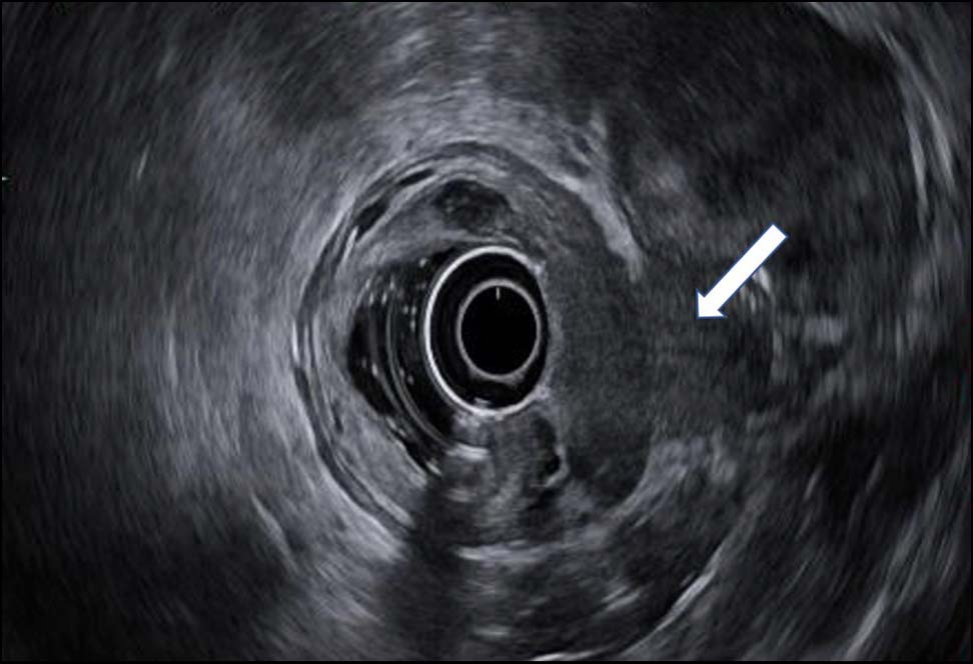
Mass (white arrow) abutting the rectum and prostate on endoscopic ultrasound.
